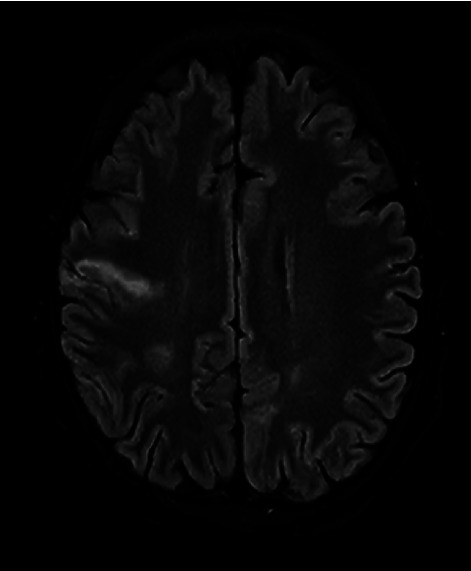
Magnetic resonance imaging (MRI) of the brain on the other hand showed focal gyriform enhancement along the right central sulcus (Rolando's sulcus) with adjacent parenchymal oedema suggestive of cerebritis.
